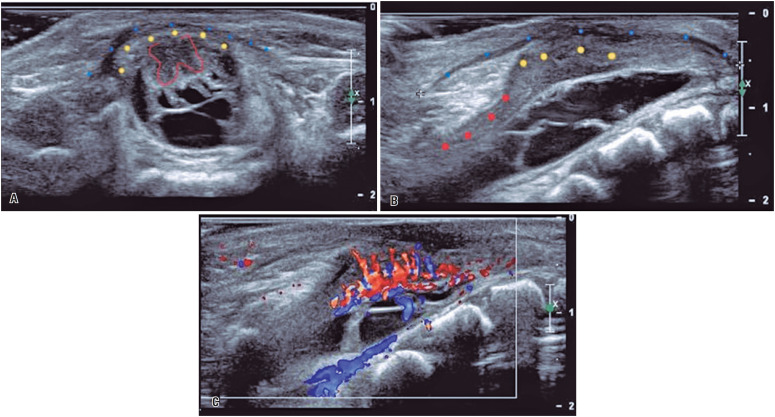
Postnatal ultrasound of the spinal column at the level of the spina bifida repair. (A) Transverse section shows the biocellulose patch (blue dots), the possible neoduramater (yellow dots), and the red line depicts the preserved anterior horns. (B) Longitudinal section showing the biocellulose patch (blue dots), the former placode (yellow dots), and the normal image of the medulla reaching the repaired area from the cranial direction (red dots). (C) At the same level, the color Doppler shows the intense vascularization of the former placode. Note: the vessels do not cross the biocellulose patch
